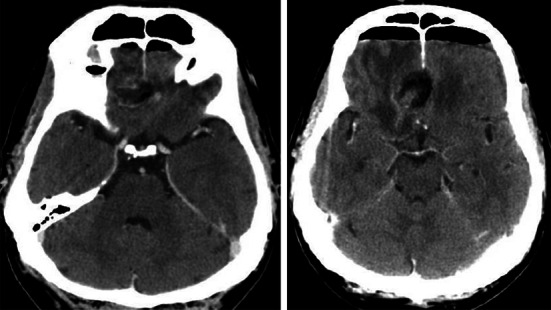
Case 2—follow up CT scan
